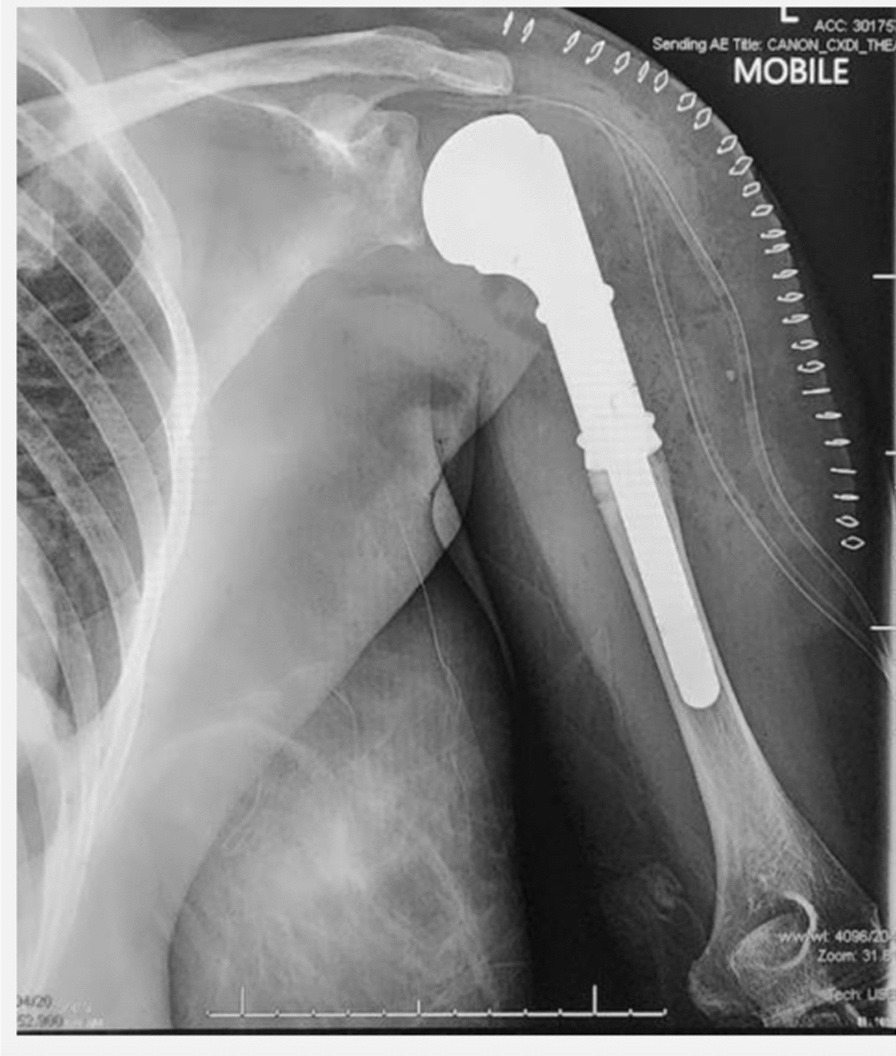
Post-operative AP and Lateral radiographs of the left shoulder after revision arthroplasty
